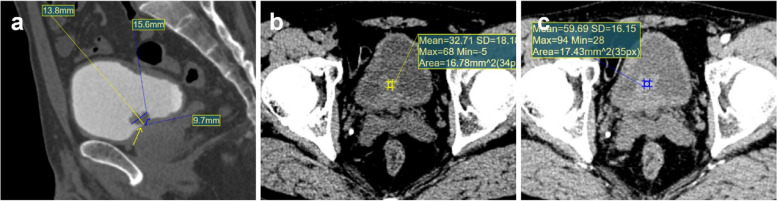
A 54-year-old male patient who underwent TURBT and was confirmed as Bladder inverted papilloma. a Sagittal reconstruction excretory phase image showed the lesion was located at the Bottom wall, the junction between lesion edge and bladder wall was smooth (arrow), the maximum transverse diameter of this lesion was 1.56cm, the maximum longitudinal diameter was 1.38cm (Transverse diameter/longitudinal diameter=1.13 ) and the tumor-wall interface was 0.97cm (yellow curve). b The non-enhanced CT value of this lesion was 32.71HU. c The arterial phase CT value of this lesion was 59.69HU, the arterial reinforcement rate was 0.82 ([59.69-32.71]/32.71)
